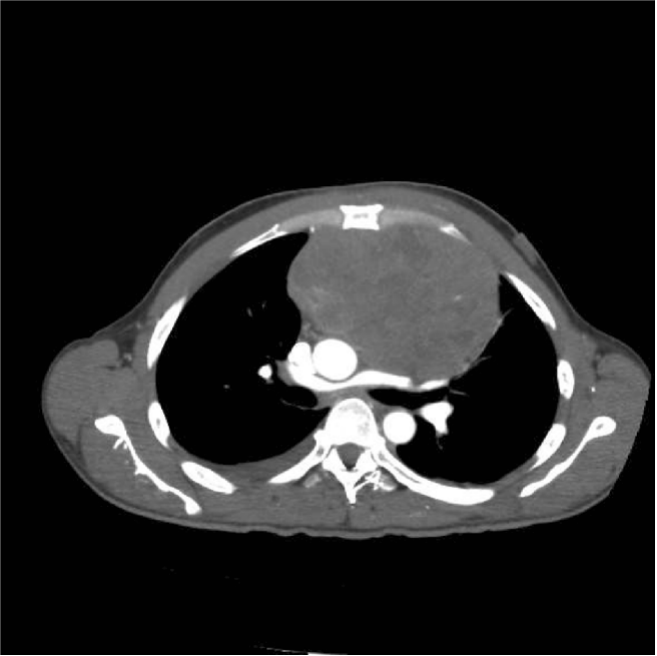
Chest CT scan showing a mediastinal mass.
